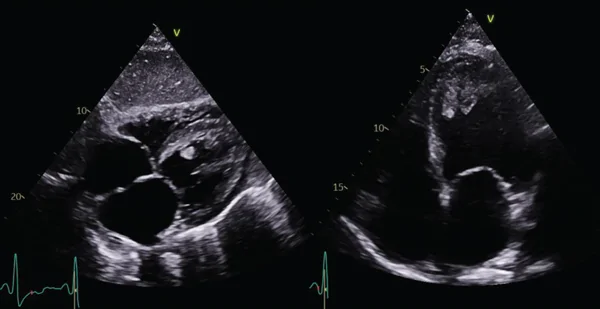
Four‐chamber view transthoracic echocardiogram on Day 2 of admission.
